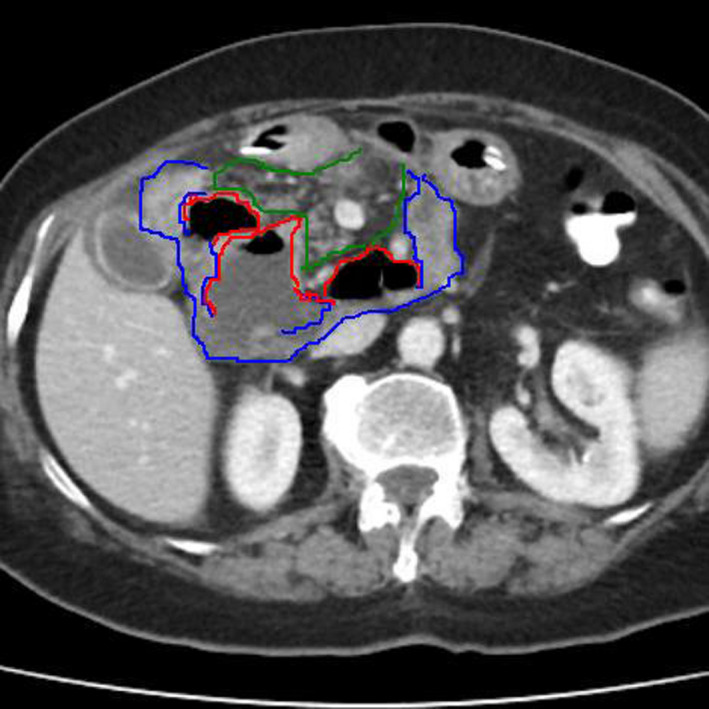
CT review revealed three duodenal diverticula depicted as three ill‐defined, air‐containing, mass‐like, and abscess‐like lesions (red outline) between the duodenum (blue outline) and pancreas (green outline)
